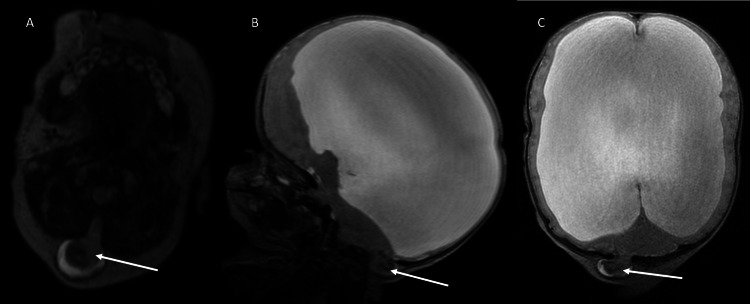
Occipital Encephalocele Axial (A), sagittal (B), and coronal (C) T2-weighted images of the brain MRI performed on Day 1 of life show cerebellar tissue (arrow) extending into the occipital cephalocele. Dilated lateral ventricles are also seen.
